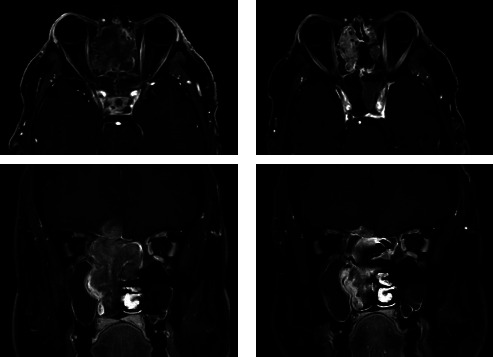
Axial and coronal postcontrast T1-weighted with fat saturation MR images of patient no. 1 with T4bN0M0 TCS located in the right nasal cavity and paranasal sinuses with contralateral extension. Note: cranial extension through the cribriform plate with (limited) invasion of brain parenchyma. (a): prior to neoadjuvant chemotherapy; (b): after 2 cycles of carboplatin/etoposide.
